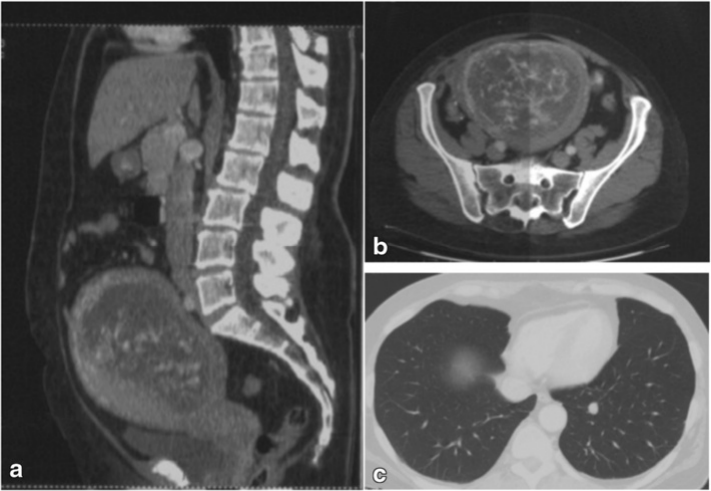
Abdominopelvic contrast-enhanced CT scans showing an enlarged uterus with focal areas of hypoattenuation; a mid sagittal plane; b transverse plan. c Axial chest CT scan (lung window) showing at least one left pulmonary metastase
